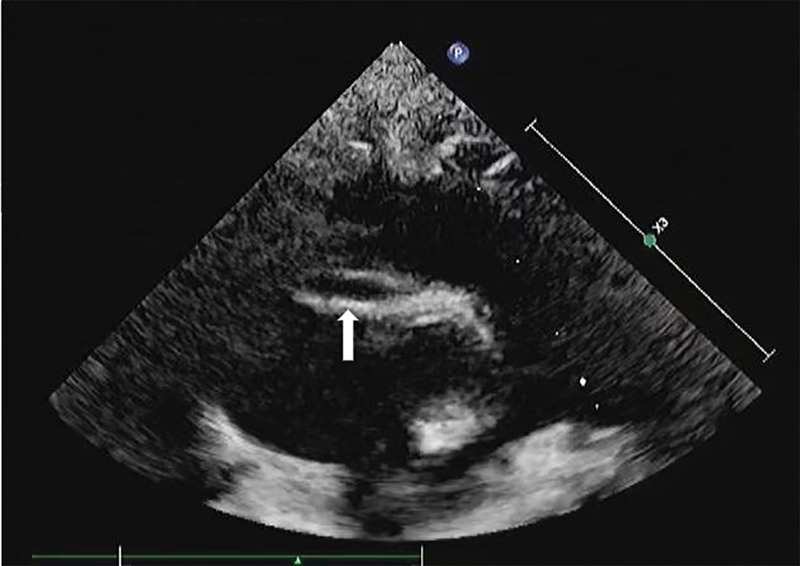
The echocardiographic image showing the right main coronary artery aneurysm (2.7 mm in diameter; *Z* score 4.06) (the arrow).
